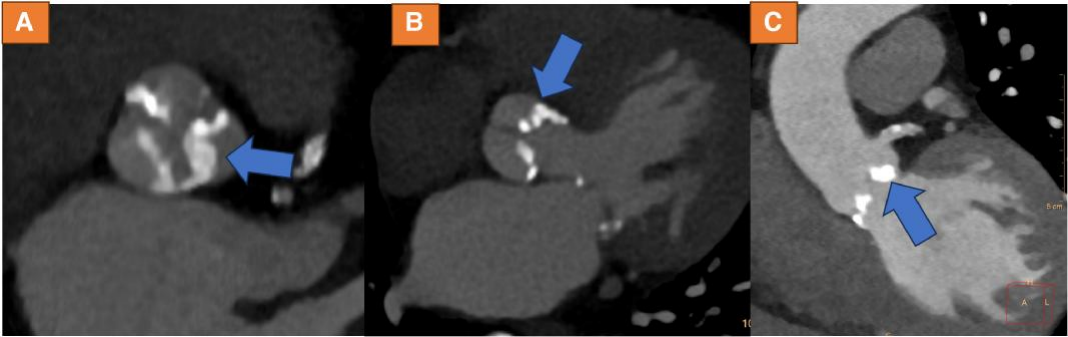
Cardiac computed tomography angiography images before transcatheter aortic valve implantation, demonstrating strong calcification of the left coronary cusp, which possibly shifted towards the left main coronary artery ostium during transcatheter aortic valve implantation (arrows in *A*–*C*).
